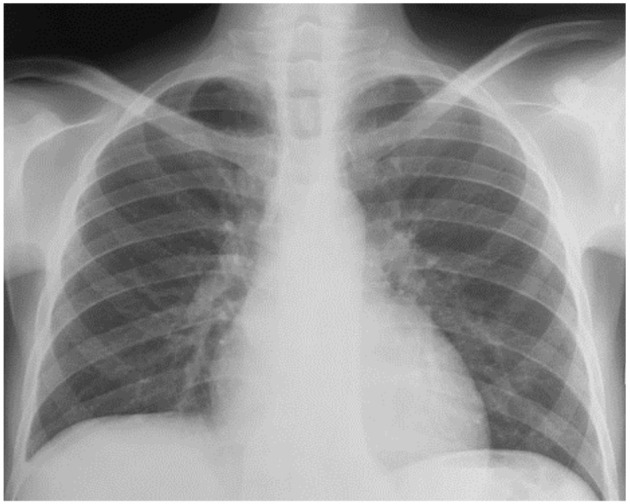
Normal initial Chest radiograph
